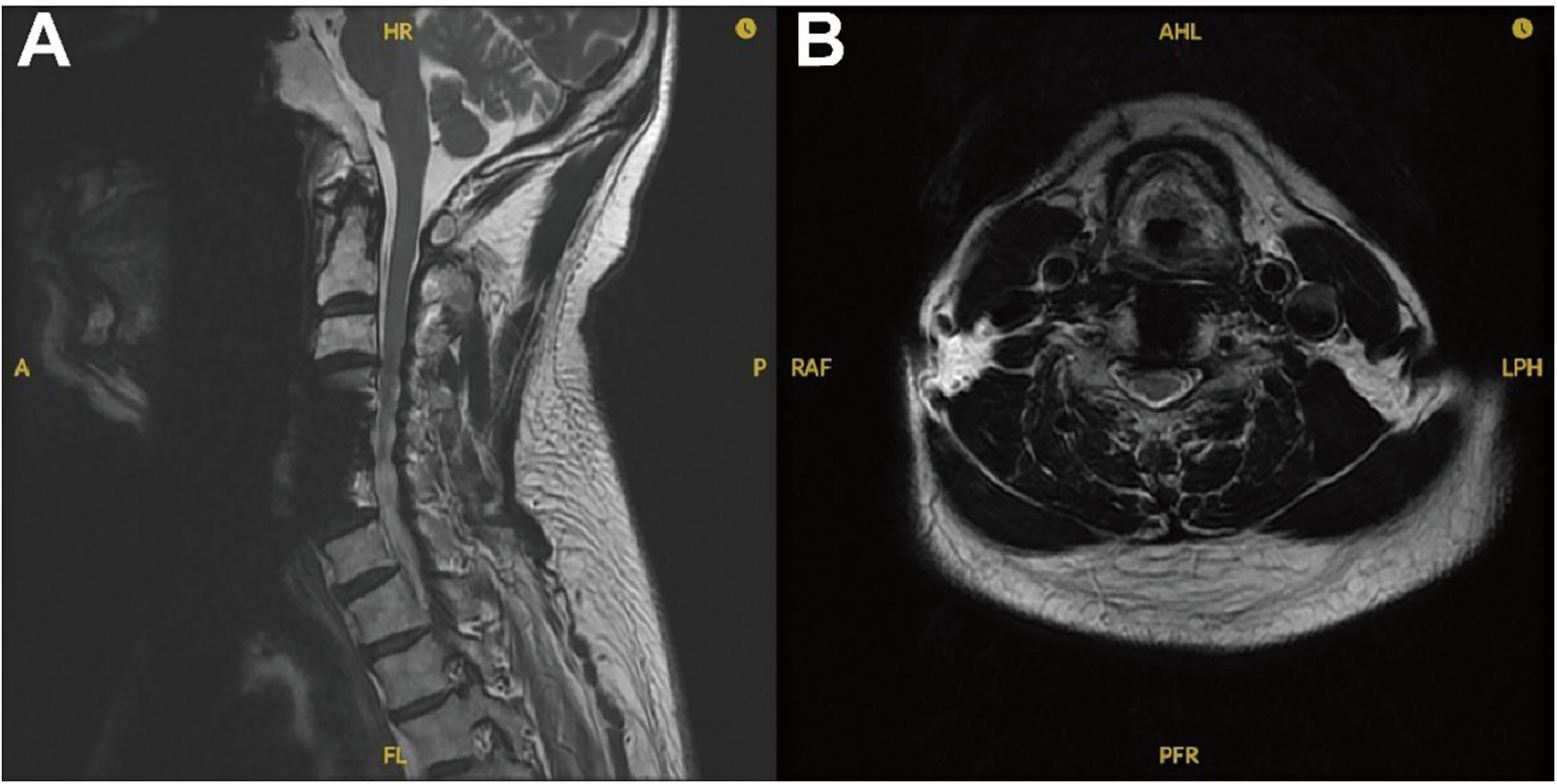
MRI at 3-month follow-up. (A) Sagittal and (B) axial T2-weighted images show the postoperative status following ACCF. The cerebrospinal fluid line is restored, and spinal cord compression has resolved.
